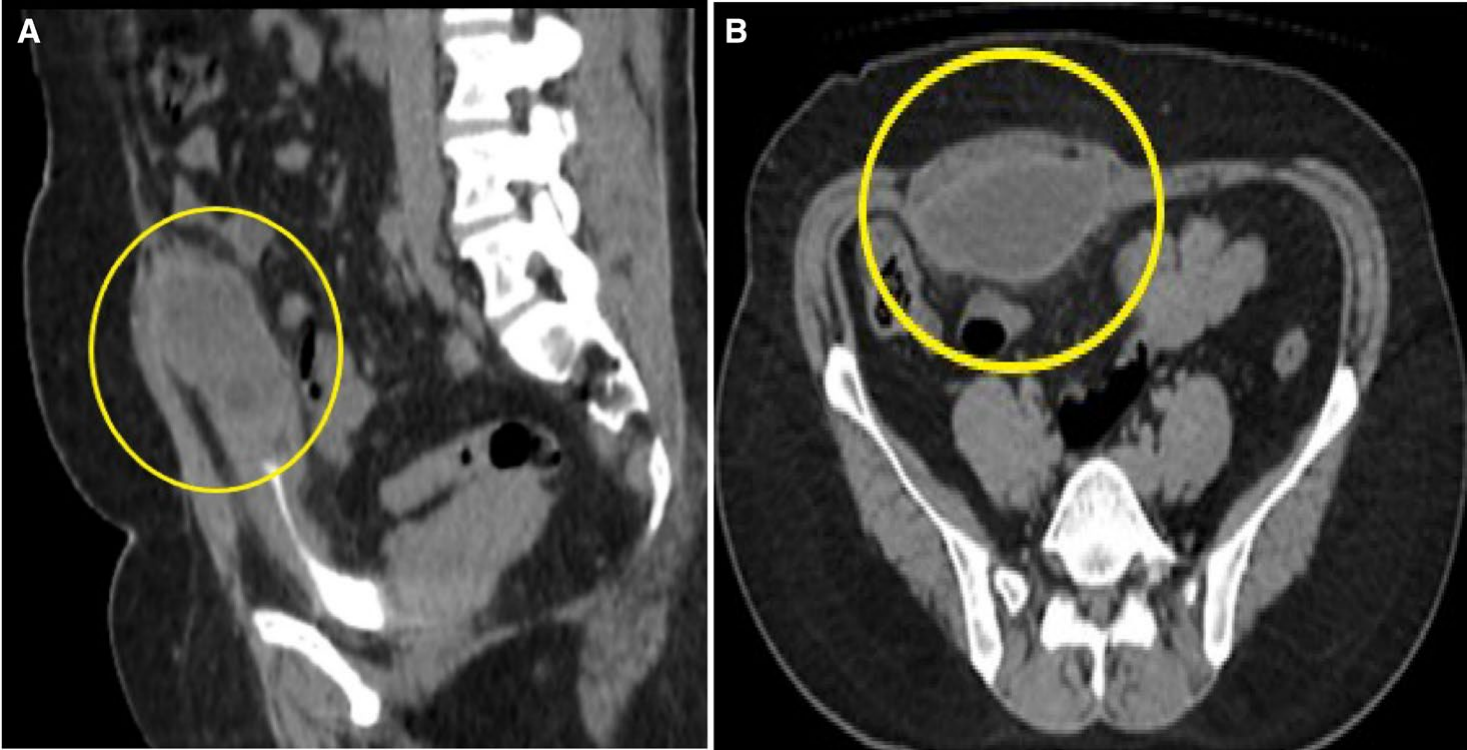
CT‐scan abdomen showing a hypodense lesion beneath the anterior abdominal wall extending up to the dome of the urinary bladder on the right side without any fistulous connection with the bladder or skin. (A) Lateral view; (B) transverse (axial) view.
